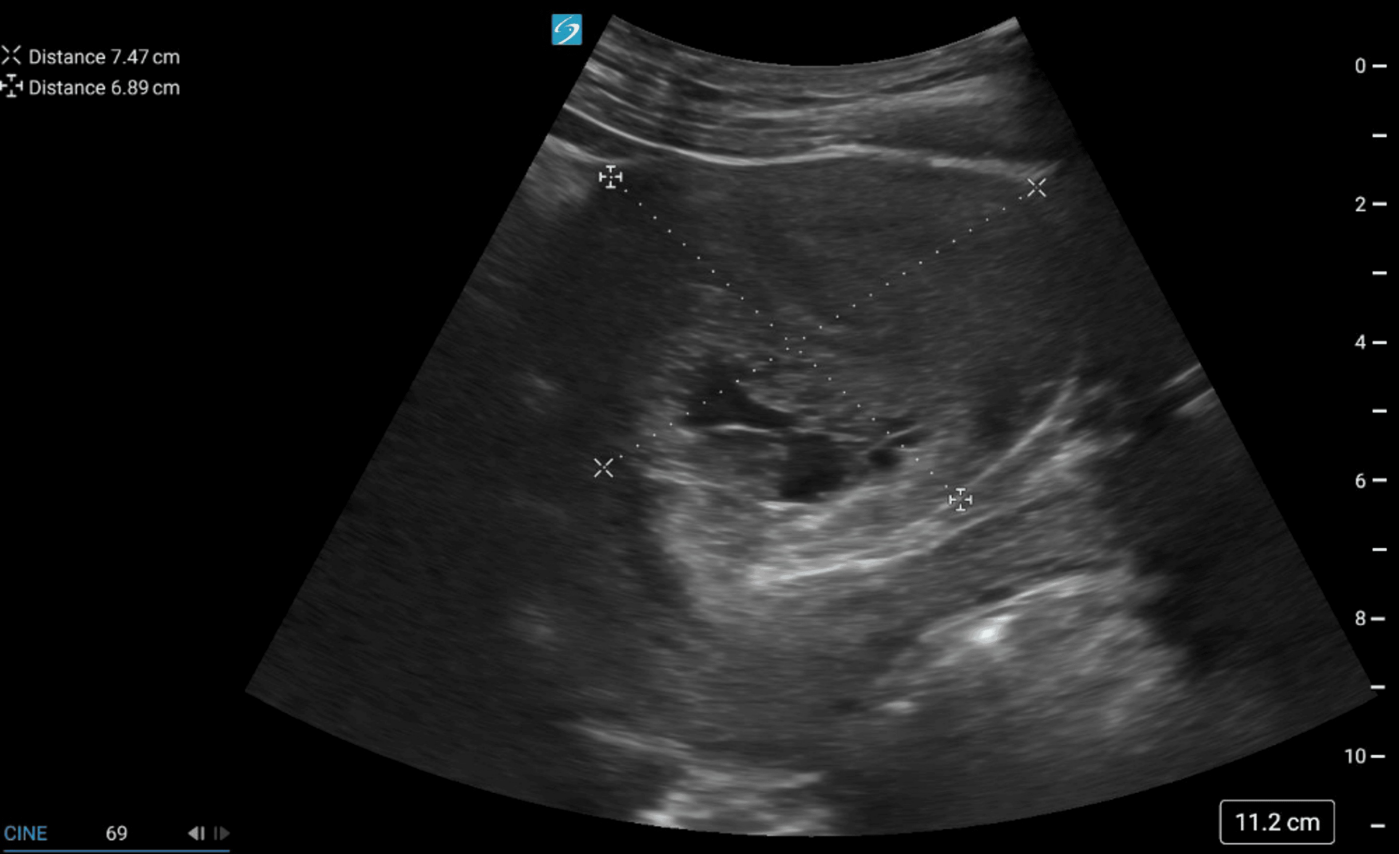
POCUS revealing an irregular complex echogenic mass within the uterus and anechoic areas with cystic structures POCUS: Point-of-care ultrasound
